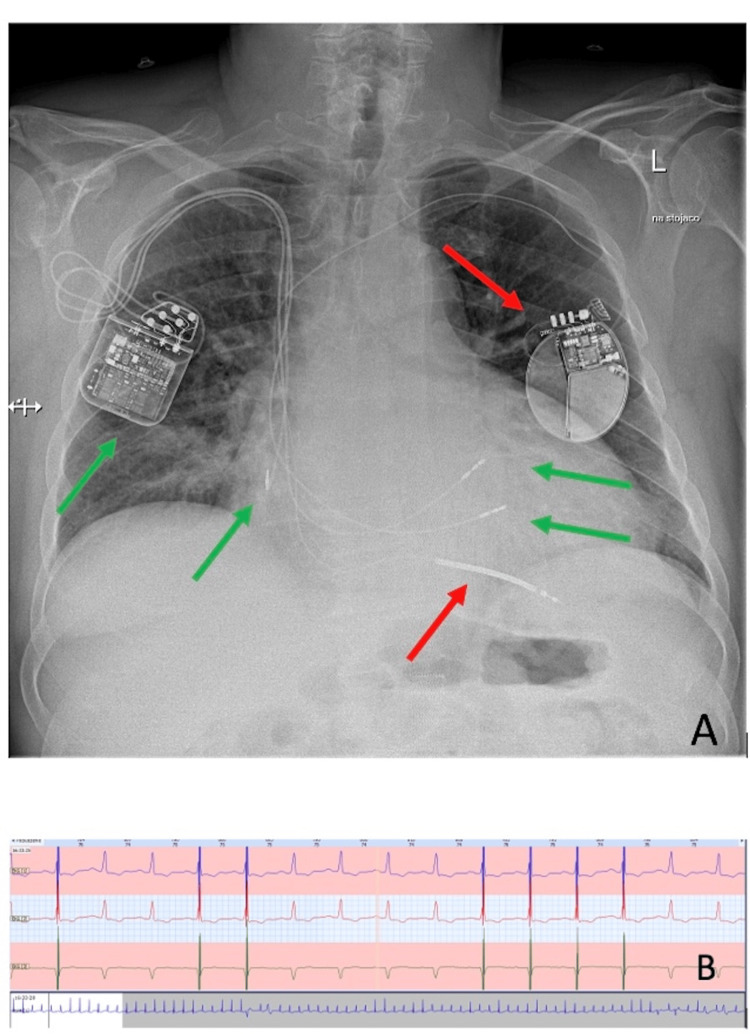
A. Chest radiograph: implantable cardioverter-defibrillator (ICD) with an ICD lead (red arrows) and the cardiac contractility modulation device with leads (green arrows). B. Holter monitoring: loss of cardiac contractility modulation.
